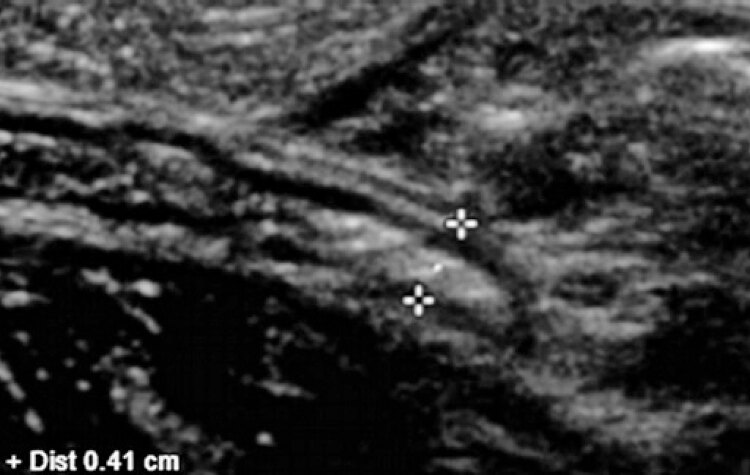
Normal cecal appendix - ultrasound measurement of cecal appendix diameter (longitudinal section)
